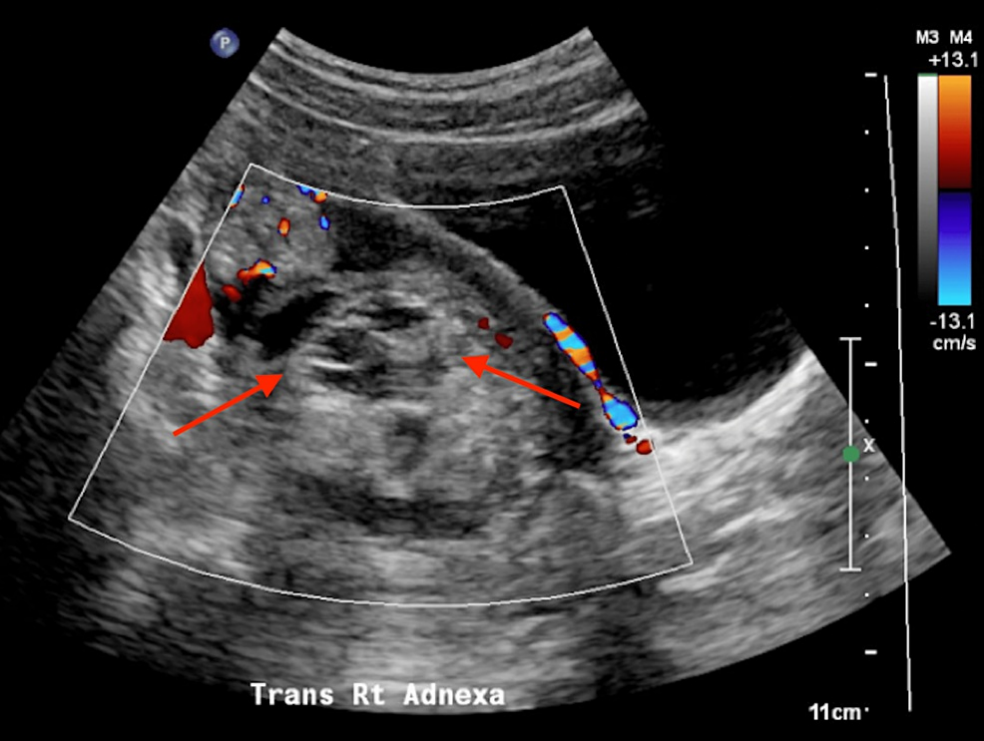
Transverse view of the pelvic ultrasound showing the heterogeneously echogenic region (red arrows) within the right adnexa without internal vascular flow.
